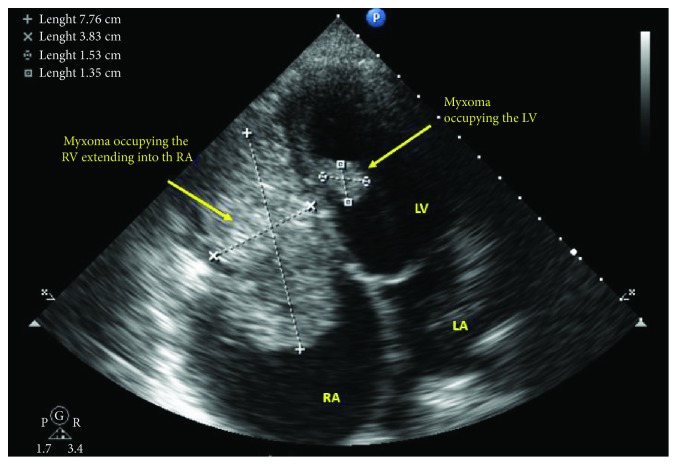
Transthoracic echocardiogram modified apical 4-chamber view showing a large mass in the right ventricle and atrium measuring 7.7 × 3.8 cm and another mass in the left ventricle attached to interventricular septum measuring 1.5 × 1.3 cm.
